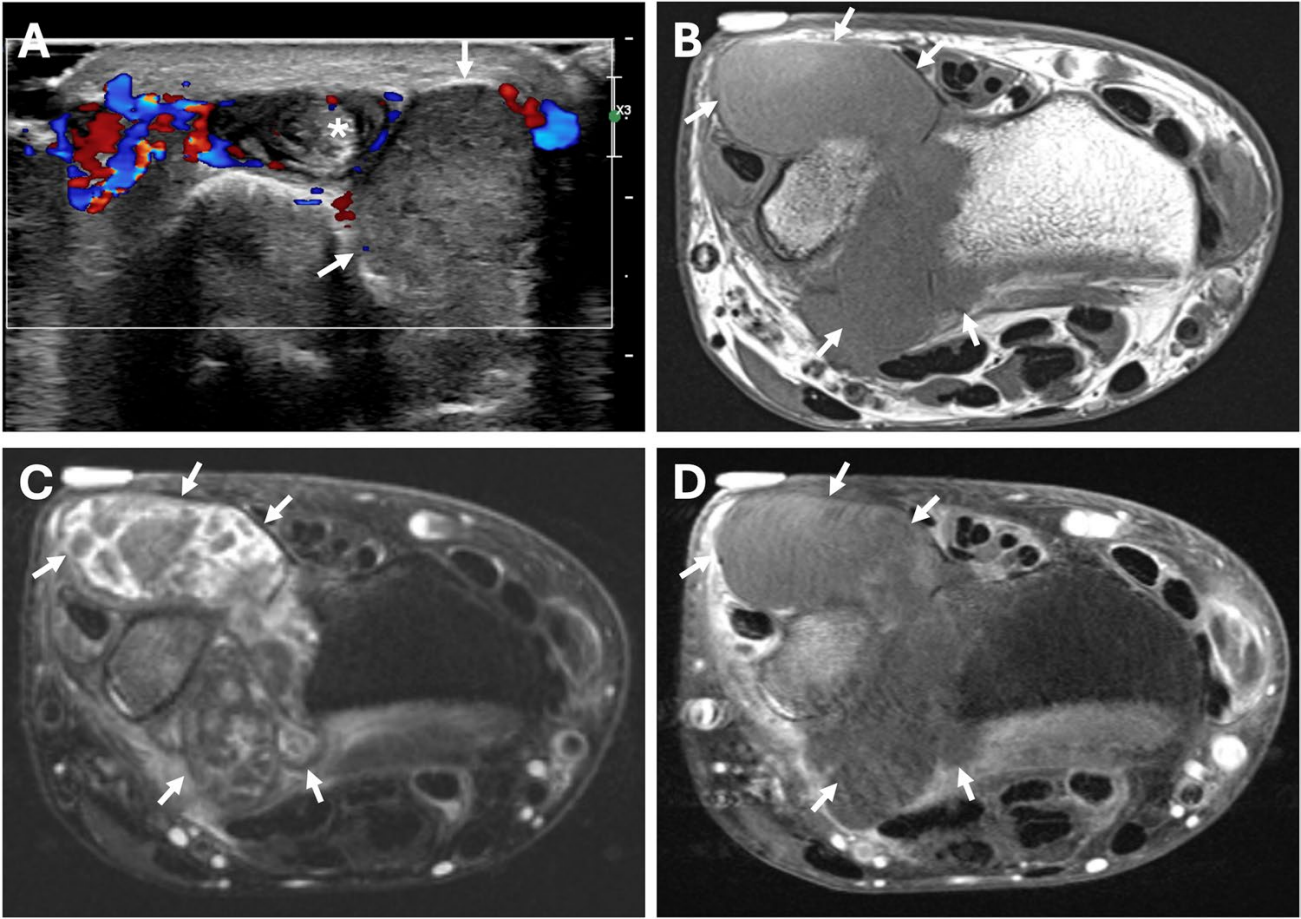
US and MR imaging of the right wrist. (A) Short axis image of the dorsal-ulnar aspect of the wrist shows iso-echoic, predominantly hypovascular mass-like distension of the dorsal recess of the distal radioulnar joint (arrows). There is also synovial proliferation within the extensor carpi ulnaris tendon sheath surrounding the tendon (asterisk). Axial (B) T1-weighted, (C) T2-weighted fat-suppressed, and (D) post-contrast T1-weighted fat-suppressed MR images show marked distension of the distal radioulnar joint (arrows) with variably sized, relatively hypointense and hypoenhancing masses and nodules
